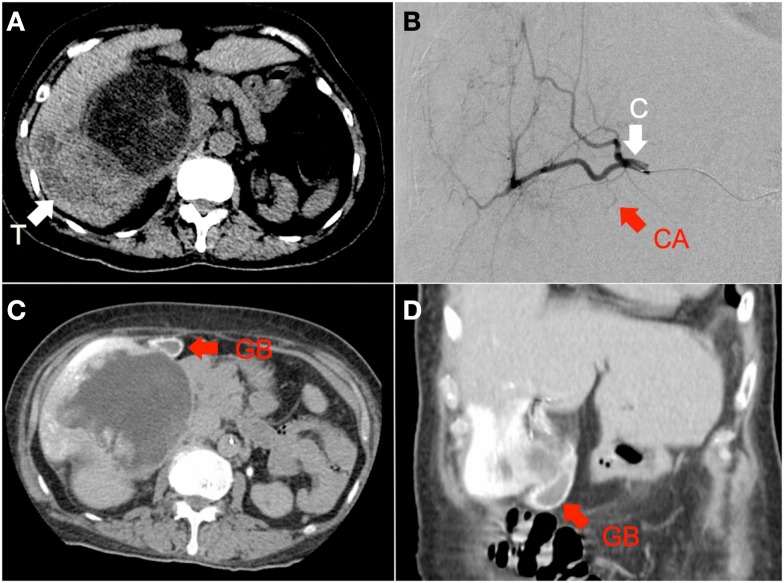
(A) Hepatocellular carcinoma of the right lobe (“T”) in a background of polycystic kidney and liver disease, seen on non-contrast-enhanced CT. (B) RE was performed with the catheter tip (“C”) in the right hepatic artery, proximal to the origin of the cystic artery (“CA”), seen here on digital subtraction angiography (C,D) Catheter-directed CT hepatic angiogram of the right hepatic artery demonstrates prominent contrast enhancement of the gallbladder wall (“GB”).
